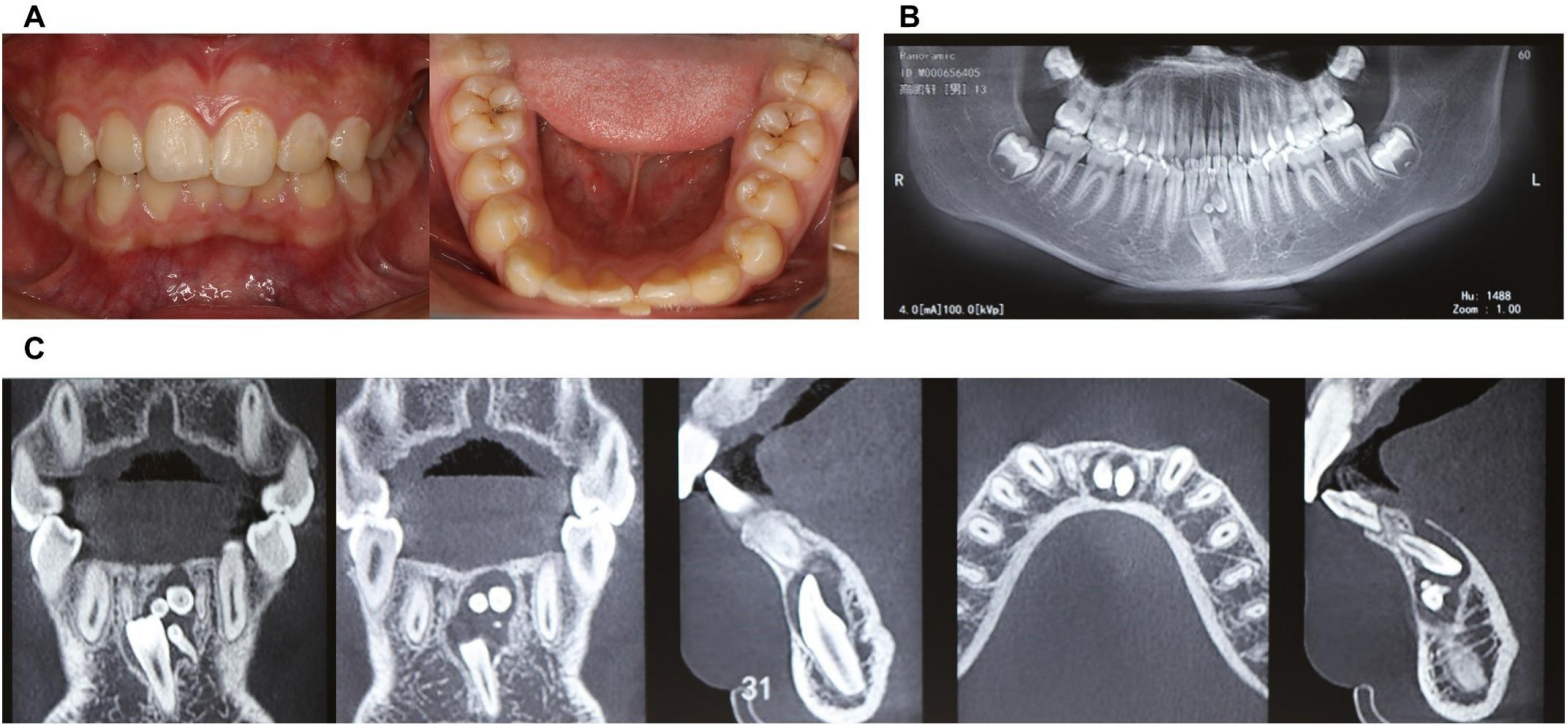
Physical and CBCT examination of compound odontoma. (A) Photos of intraoral view; (B) CBCT examination (overall view); (C) CBCT examination (location of compound odontoma in the anterior mandibular region, including sagittal, coronal, and axial planes).
